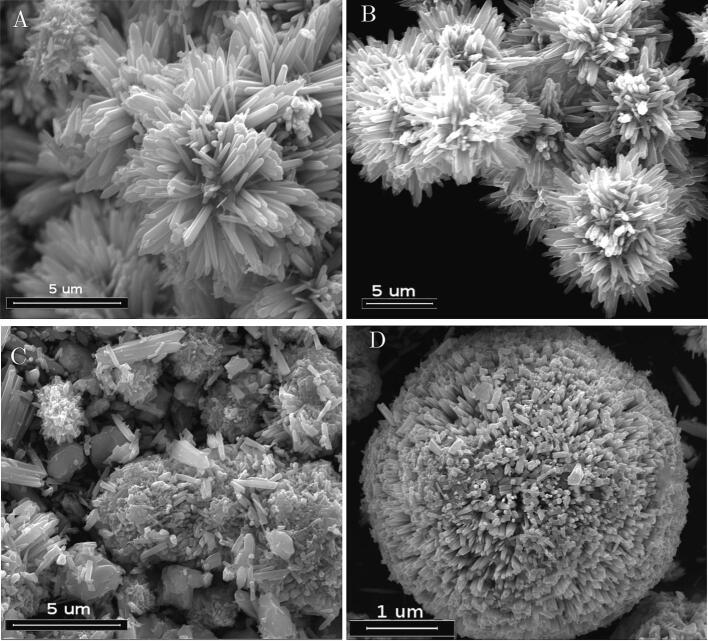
SEM investigations of ZnO-mp (multipods) particles after 90 min of ultrasonic treatment 20 kHz: A and B images of as made particles; C large view of the eroded particles with smaller residuals; D close view of a particles in which the needles composing the structured surface were completely ripped off.
